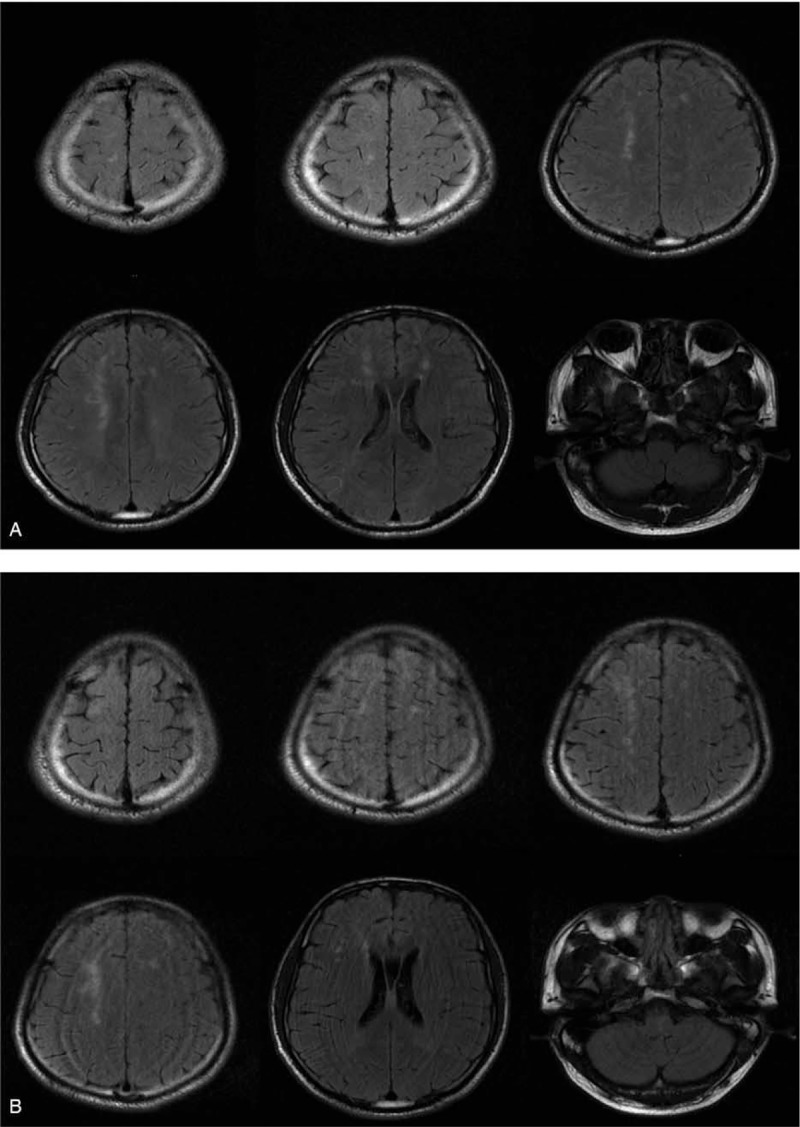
Magnetic resonance imaging (MRI) of a young male with neurosyphilis. MRI revealed (A) multiple plaques of equal-length T2 signal in the bilateral frontal lobes, centrum semiovale, and lateral ventricle, along with hyperintense FLAIR signals, and (B) numerous spots and plaques of equal-length T2 signal in the bilateral frontal lobes, centrum semiovale, right lateral ventricle, and pons, along with hyper-intense FLAIR signals.
